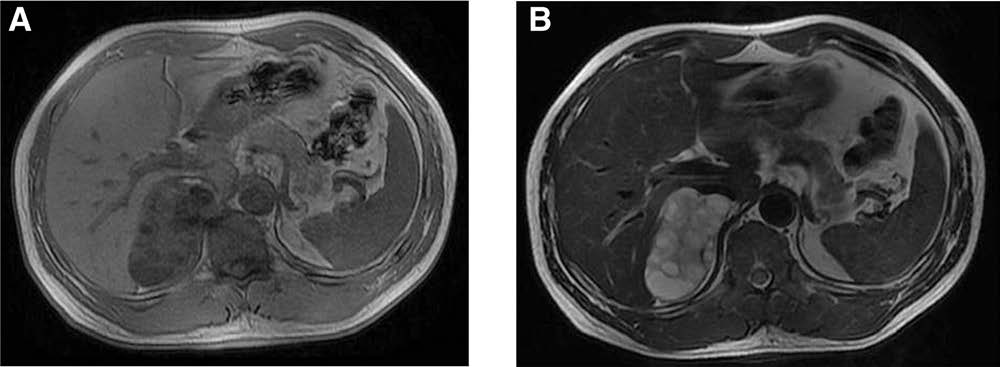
Computed tomography of the abdomen. (A and B), Computed tomography scan showing a polycystic mixed-density lesion in the right adrenal region, approximately 7.3 × 4.5 cm in size.
